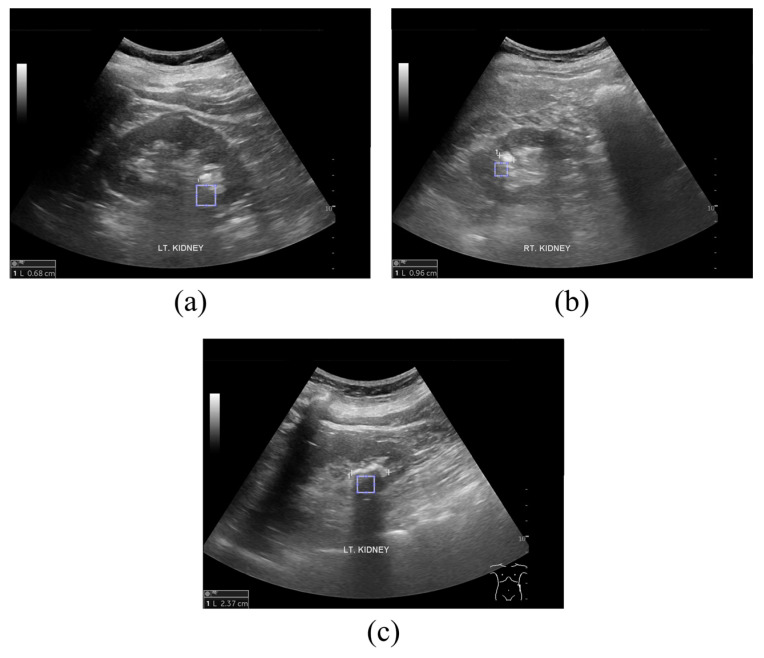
Kidney stone ultrasound images acquired for GLCM analysis. Sample ultrasound images of suspected kidney stones, with probabilities of (a) 50–60%, (b) 60–80%, and (c) ≥80%, analyzed by doctors and sonographers.
